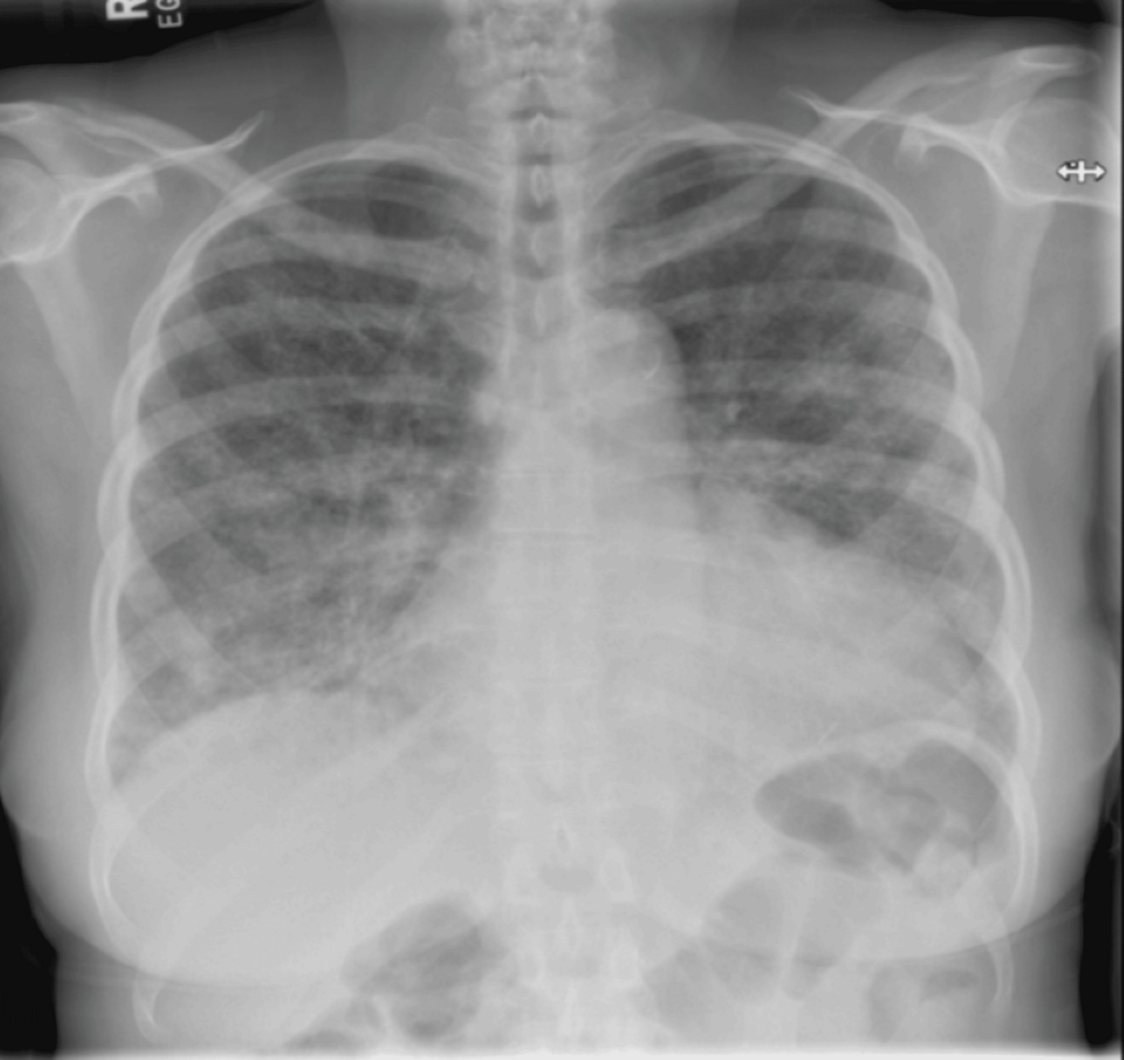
Chest X-ray on admission demonstrating bilateral patchy airspace opacities and areas of ground-glass attenuation, more prominent in the lower zones. No pleural effusion, or pneumothorax is identified. The cardiomediastinal silhouette is within normal limits. No acute osseous abnormality is seen.
